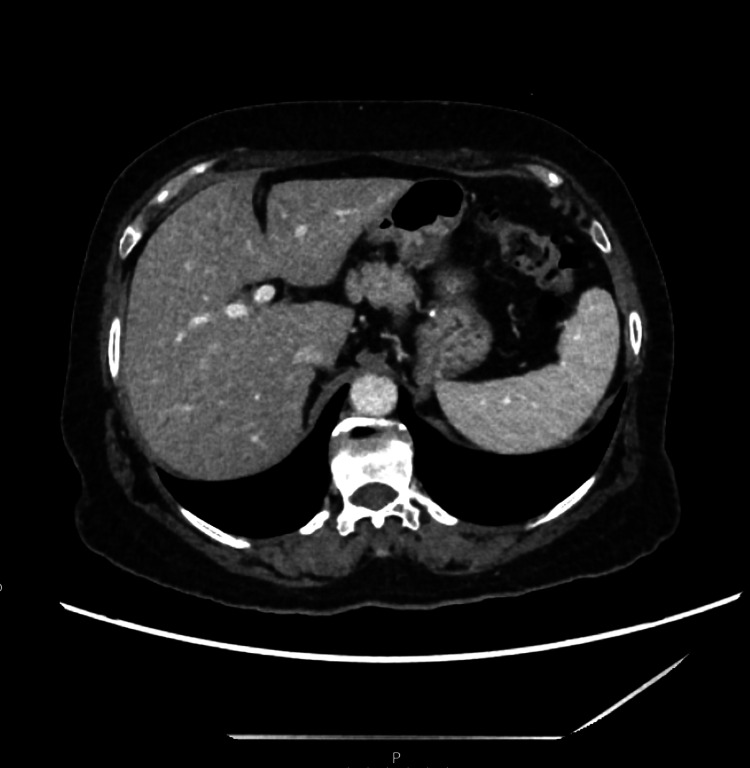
Computed tomography of the abdomen with contrast revealing no acute findings.
